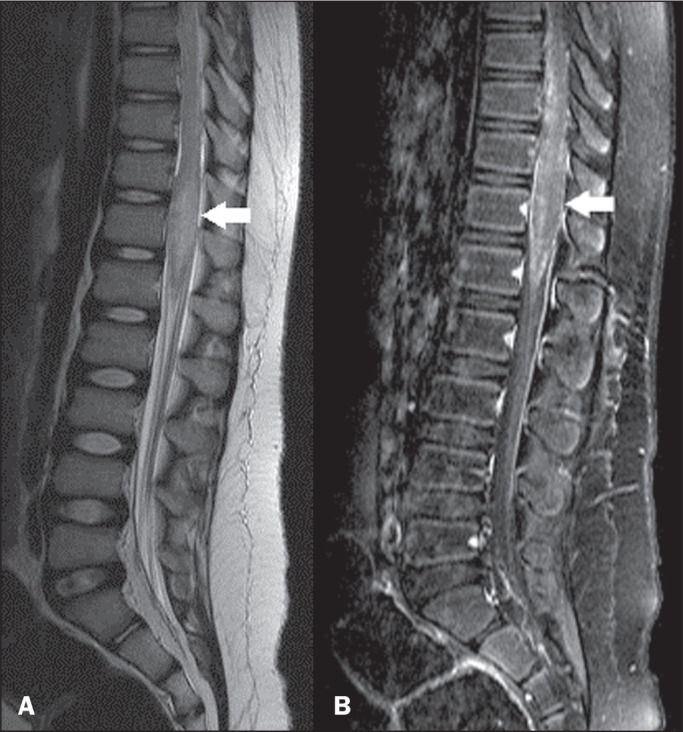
Spinal cord schistosomiasis. Sagittal T2-weighted MRI sequence (A)
